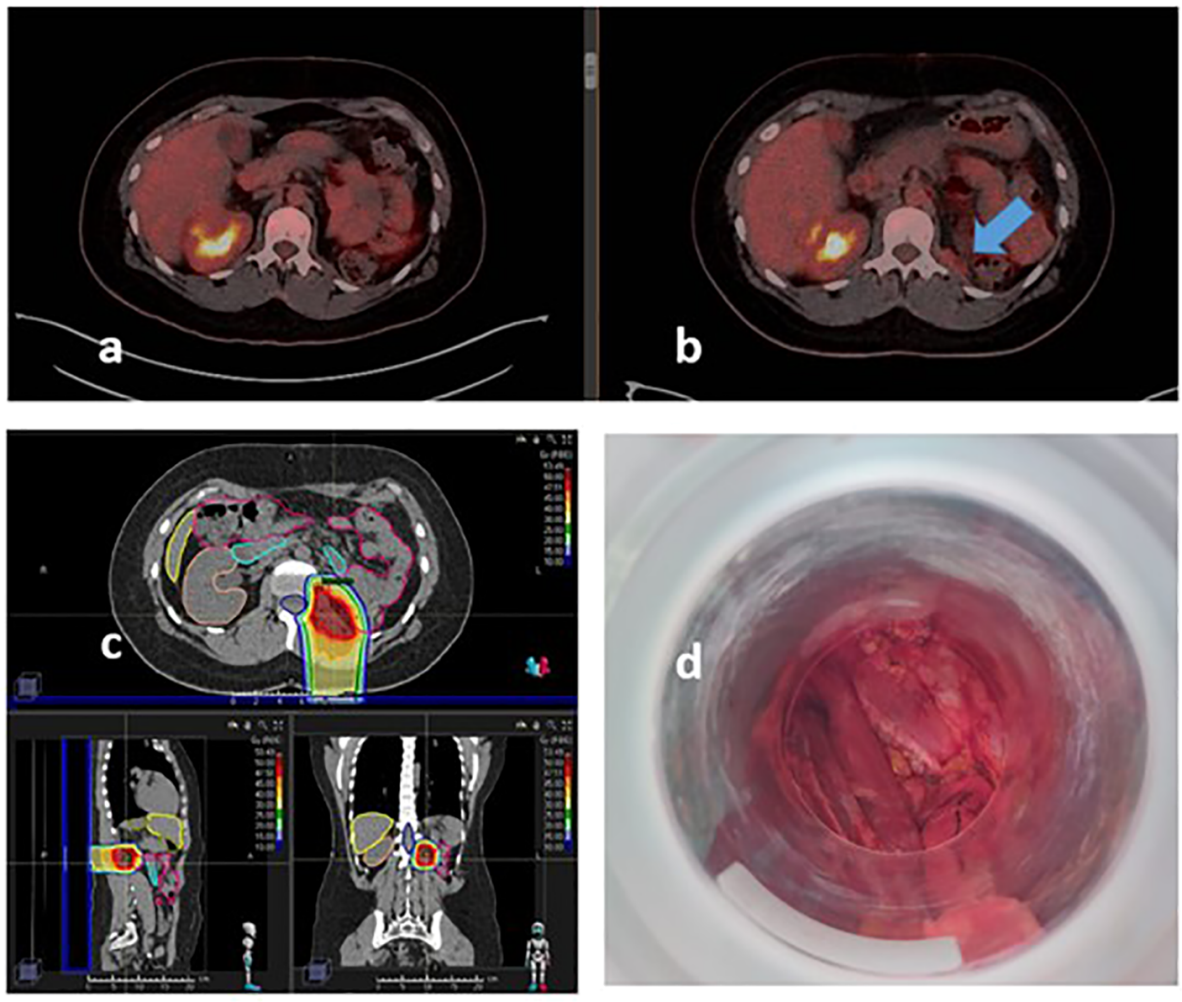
Recurrent synovial sarcoma of the lumbar fossae treated with proton therapy and IOeRT. (A) Restaging PET-CT after proton therapy. (B) Pre-proton therapy PET-CT showing metabolic active persistant disease before chemo-radiation in the retroperitoneal left lumbar fossae. (C) Single field proton beam arrangement and dose distribution. (D) Beam’s eye view of IOeRT: no mobile sensitive tissues in the target displaced mechanically.
